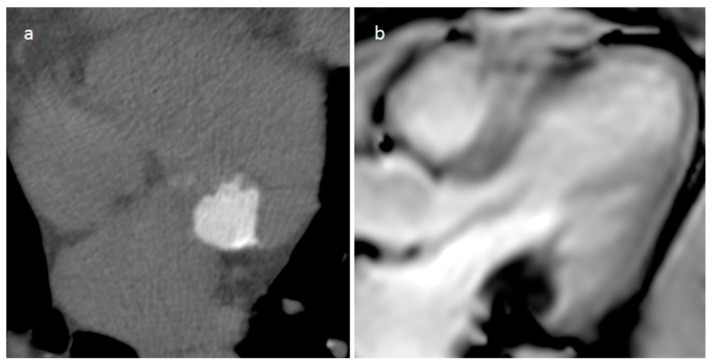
A 74-year-old female patient with mitral annular calcification on precontrast-phase CT image (a) and cine sequence (b).
